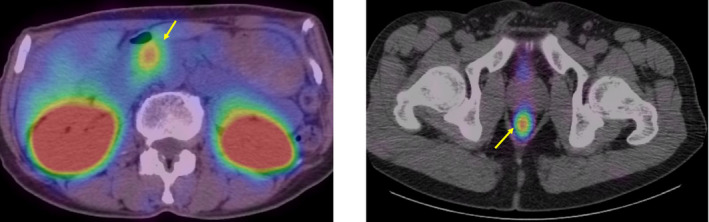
Abnormal accumulation at sites of the tumor seen on an indium‐111 (^111^In) pentetreotide somatostatin receptor scintigraphy scan (OctreoScan)
